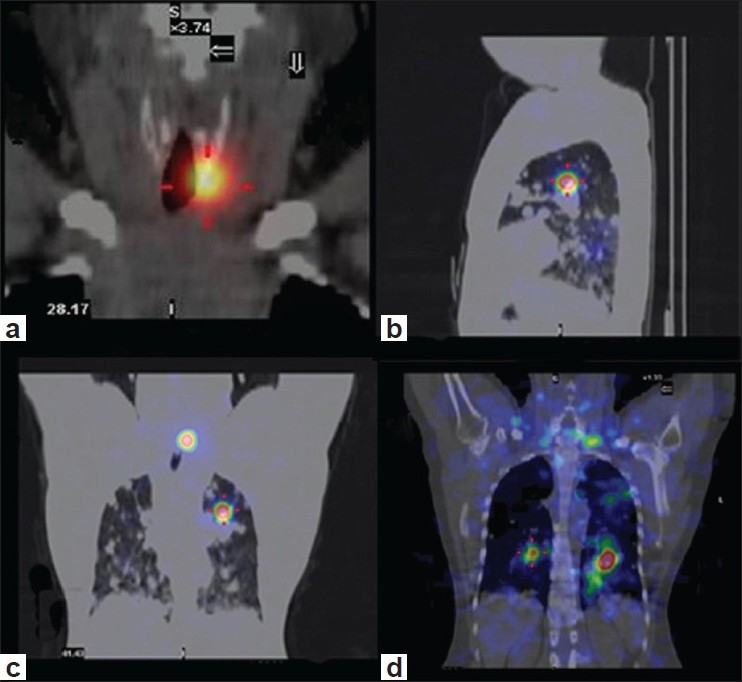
a) Hybrid SPECT-CT fusion shows I-131 uptake in the residual thyroid tissue on the left side of the neck; b-d) Multiple radio-opaque nodules are seen in both lungs, with radioiodine concentration in a few of these nodules
